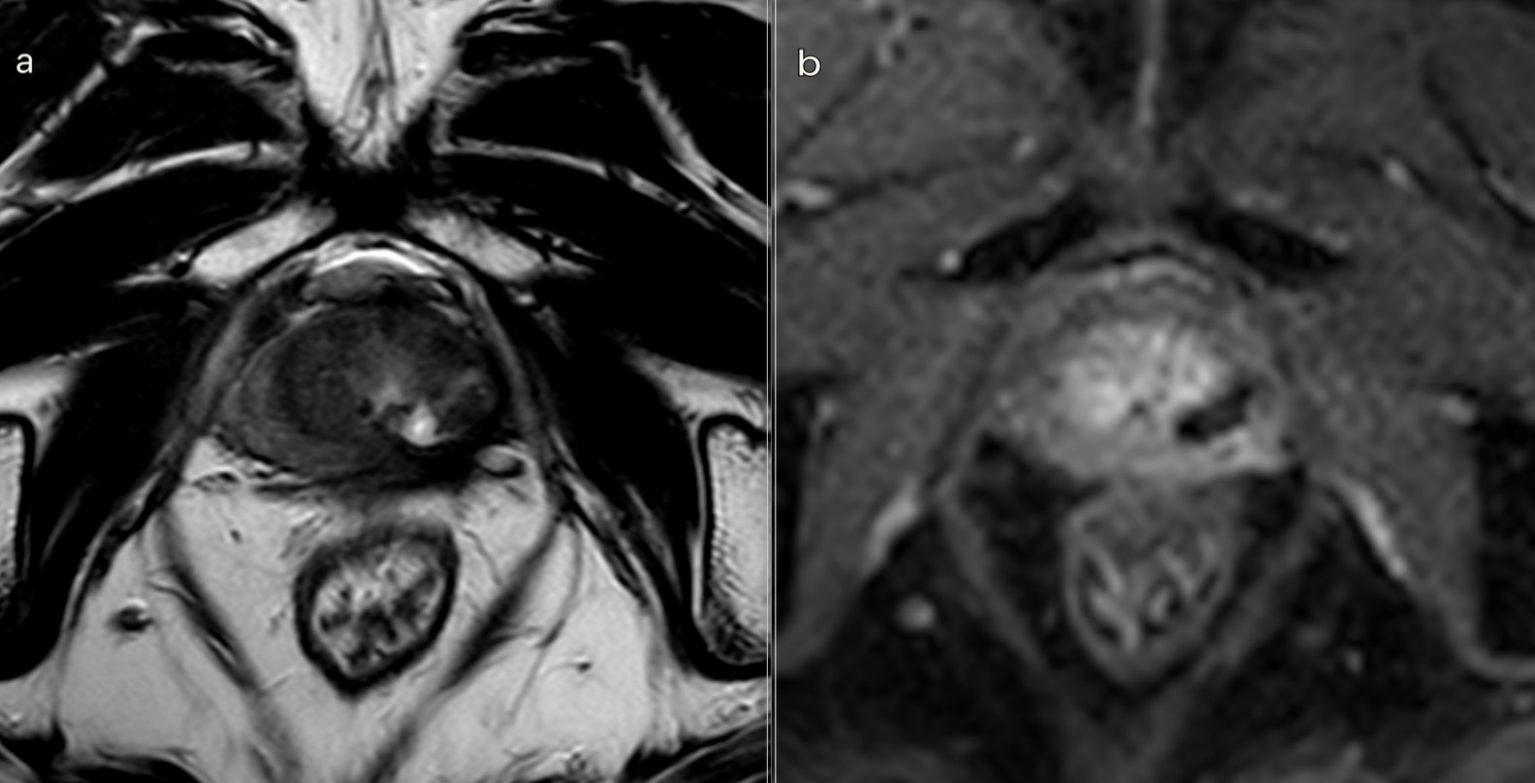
3T multiparametric MRI performed at 6 months imaging follow-up after TPLA treatment. *T*
